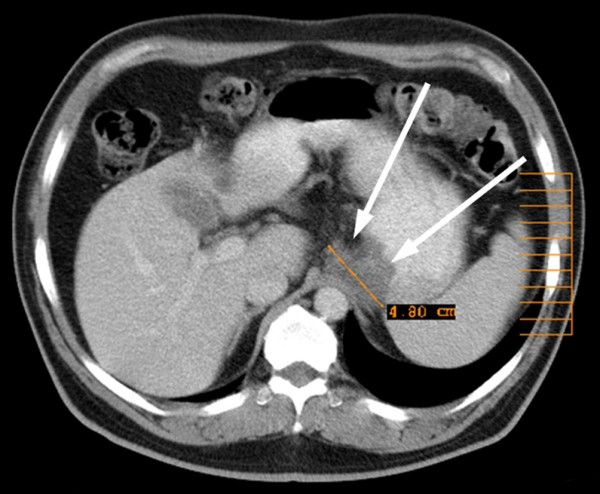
Follow up CT-Scan. Follow up CT scan after 6 months of treatment with imatinib mesylate, showing considerable regression of tumor.
